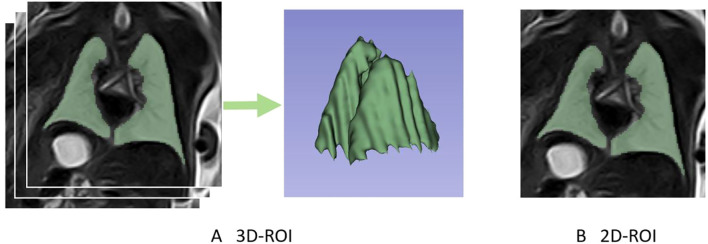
Representative coronal T2-weighted single fast spin-echo fetal lung MRI at 36 ^+ 2^ weeks of gestation. (A) 3D whole-lung ROIs generated using a semi-automated approach with manual refinement. (B) The corresponding 2D ROI, delineated in the plane of the tracheal bifurcation.
